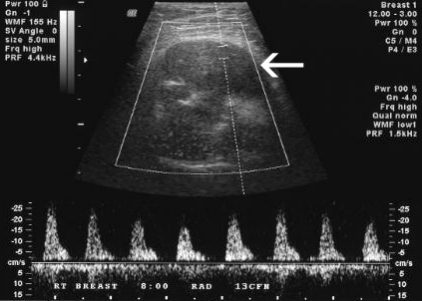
Doppler breast ultrasound demonstrates hypo-echoic solid right breast mass with internal vascularity (arrow).
